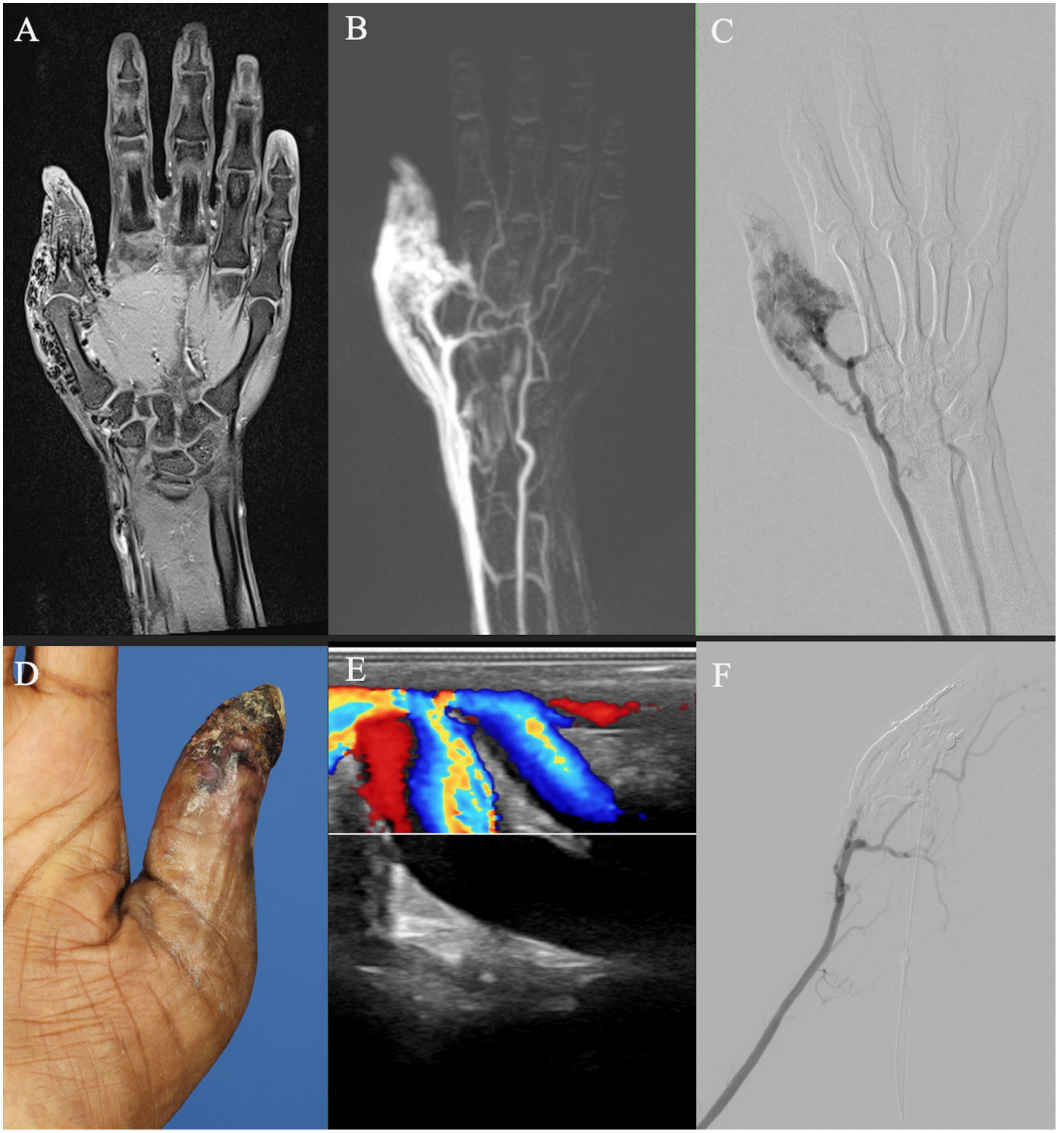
Imaging of an adult patient with an arteriovenous malformation of the right thumb. (A, B) Magnetic resonance imaging (MRI) shows a dilated vessel with flow voids on a T1 fat-saturated image after intravenous contrast agent admission (A), and a vessel convolute involves the thumb area on a time-resolved angiography with interleaved stochastic trajectories (TWIST) MR angiography with already venous contrast due to the shunt volume (B). (C) Digital subtraction angiography after arterial cubital puncture shows less arterial component than suspected on MRI. (D) Clinical photo of the patient's thumb. (E) Color Doppler ultrasound image demonstrates arterialized flow. (F) DSA after percutaneous embolization demonstrates devascularization of the AVM and earlier arterial perfusion of the palmar arch and the index finger.
